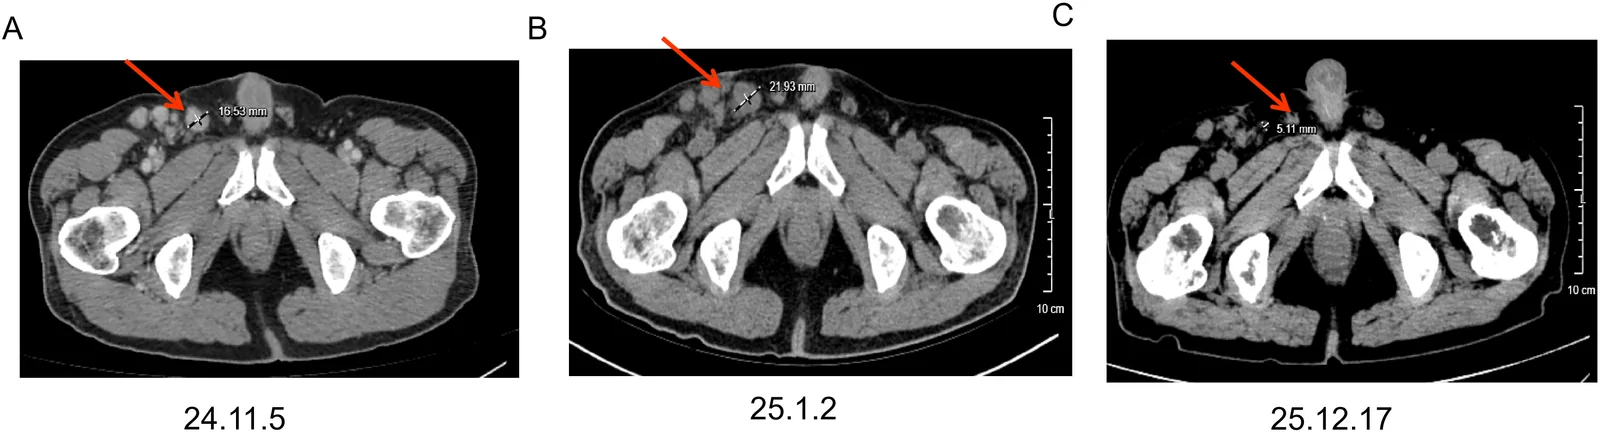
The abdominal CT scan showed multiple enlarged lymph nodes in the right inguinal, bilateral iliac vessel, right pelvic wall, and retroperitoneal regions. Compared to the abdominal CT scans presented in (A, B), which were recorded on November 4, 2024 and January 2025, the transverse diameter of one representative lymph node in the right inguinal region decreased from 16.53 mm and 21.93 mm, respectively, to 5.11 mm on December 17, 2025 (C).
